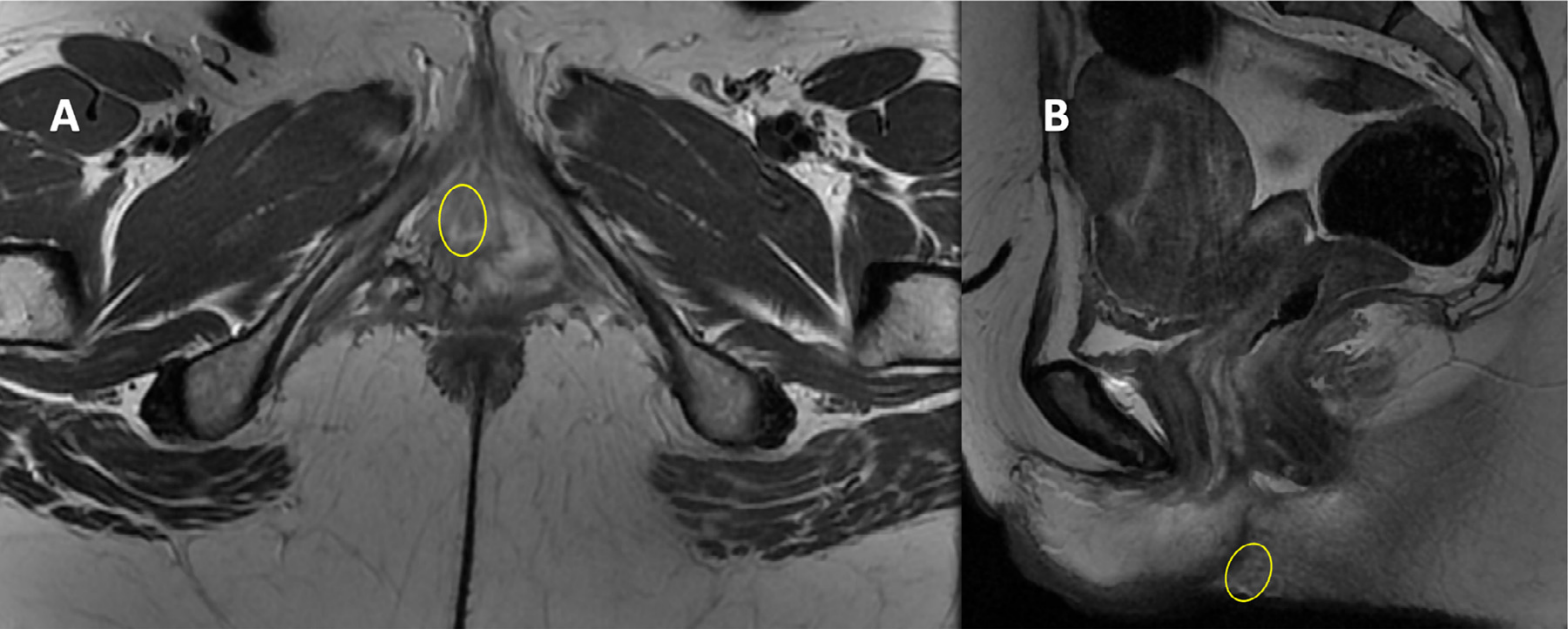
MRI T2W scan of a 39-year-old female, presenting with rapidly enlarging 1 cm vulval lump, shows FDG-avid lesion involving right vulva without invasion of the urethra, bone or muscle. June 2019, preoperative. (A, axial view; B, sagittal view).
